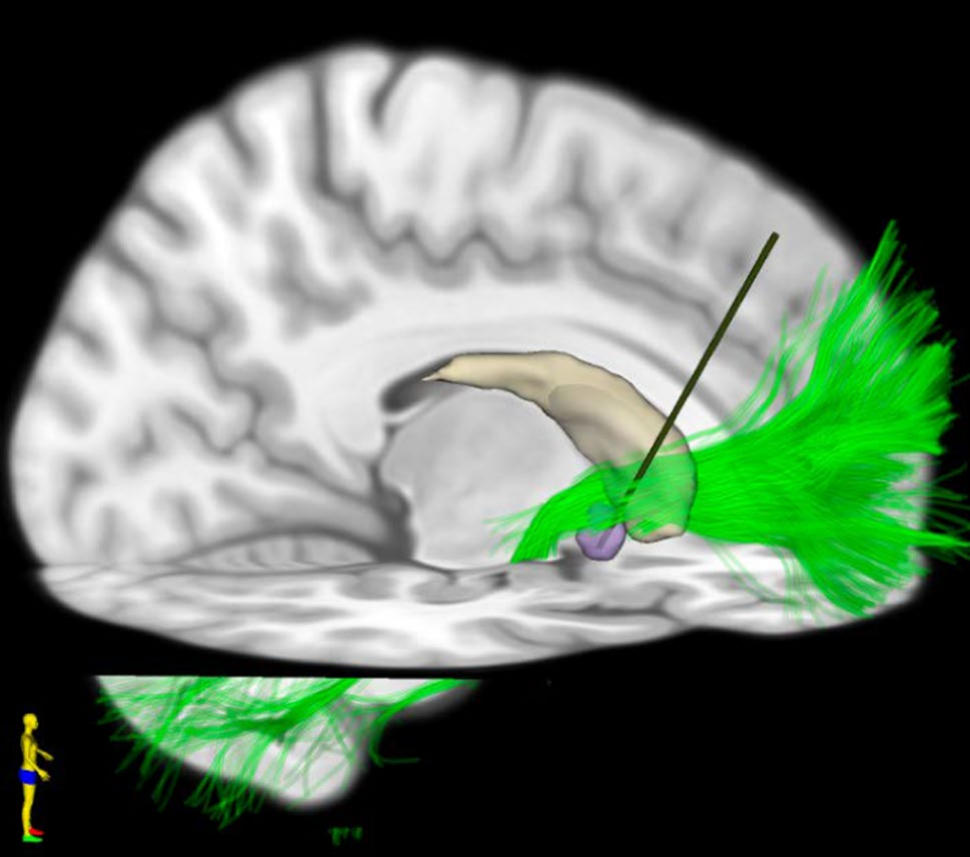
Sagittal view of a standard T1W brain MRI with a representative DBS electrode (green) traversing the anterior limb of the internal capsule (ALIC, bright green) and the nucleus accumbens (NAC, red) located ventral to the caudate (white)
